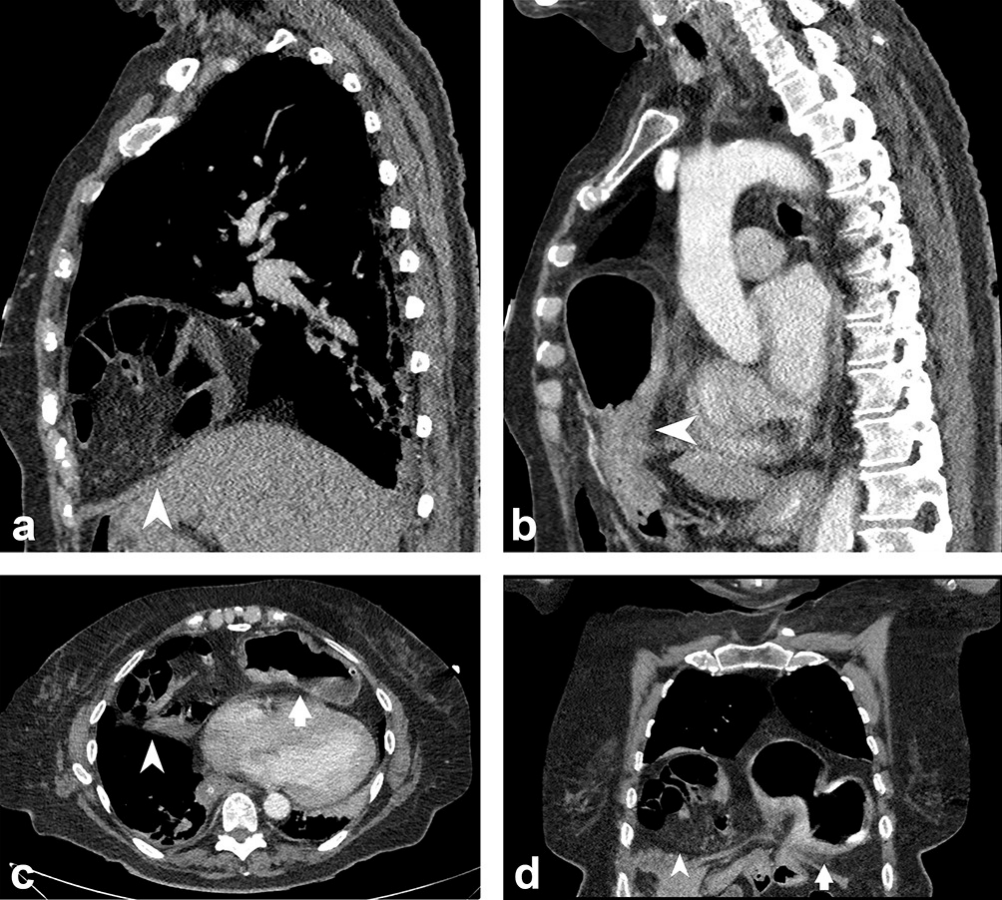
CT images demonstrating (a) herniation of small bowel and mesentery through the right diaphragmatic defect (arrowhead); (b) stomach herniating through the left defect (arrowhead). Both the right (arrowhead) and left (arrow) diaphragmatic defects are demonstrated together in (c) axial and (d) coronal planes.
